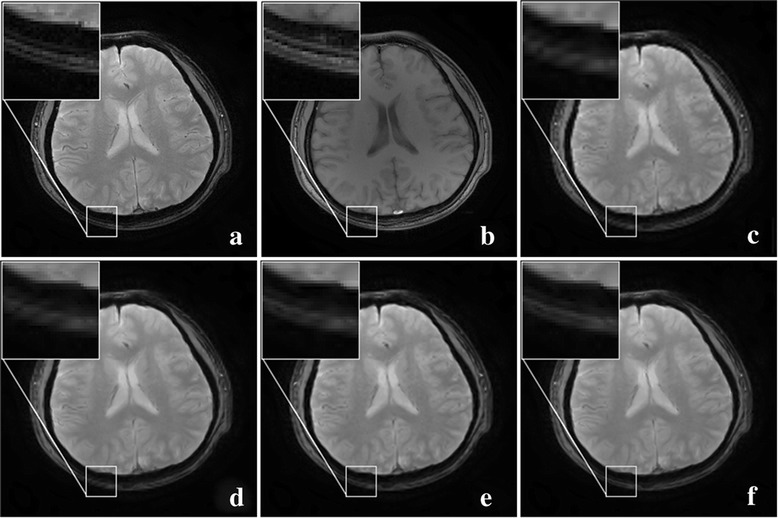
One pair of T1 and T2 MRI images acquired on 3 T Philips scanner. a HR of T2; b HR of T1; c the bicubic; d NEDI; e CGI; f the proposed method
